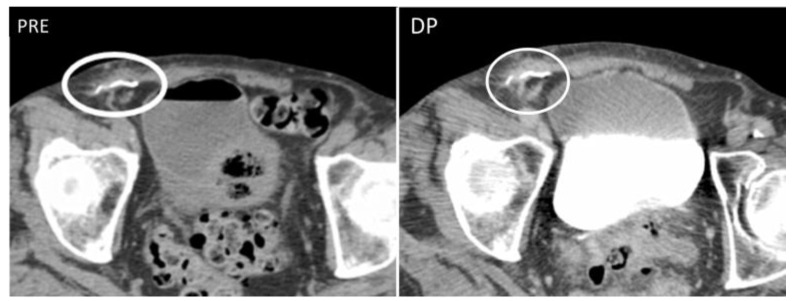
Granuloma occurring along a surgical mesh for an inguinal hernia repair. Axial CT on unenhanced (image on the left) and delayed phase at 10 min (image on the right) shows the formation of a nodular, fibrotic mass, with uptake contrast in delayed phases around a surgical mesh (circle).
